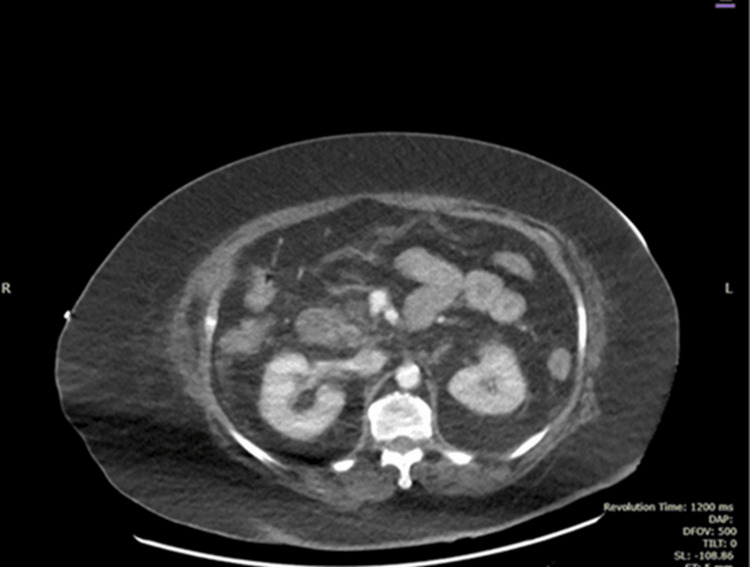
CT Abdomen with Contrast No active intestinal ischemia
